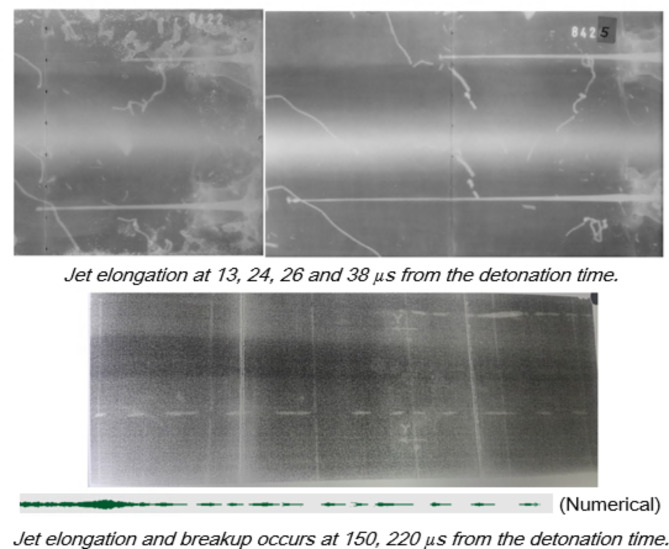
Jet profiles x-ray image at different times from the detonation moment.
